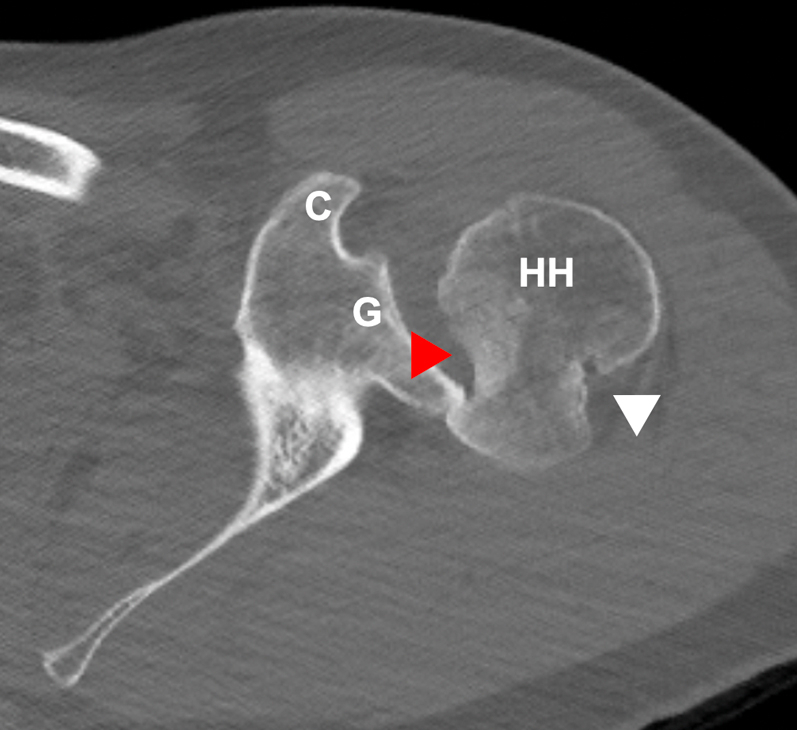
Axial computed tomography image of the patient’s left shoulder showing large Hill–Sachs (white arrowhead) and reverse Hill–Sachs lesions (red arrowhead) with the glenohumeral joint dislocated posteriorly engaging into the reverse Hill-Sachs lesion. (C, coracoid; G, glenoid; HH, humeral head.)
